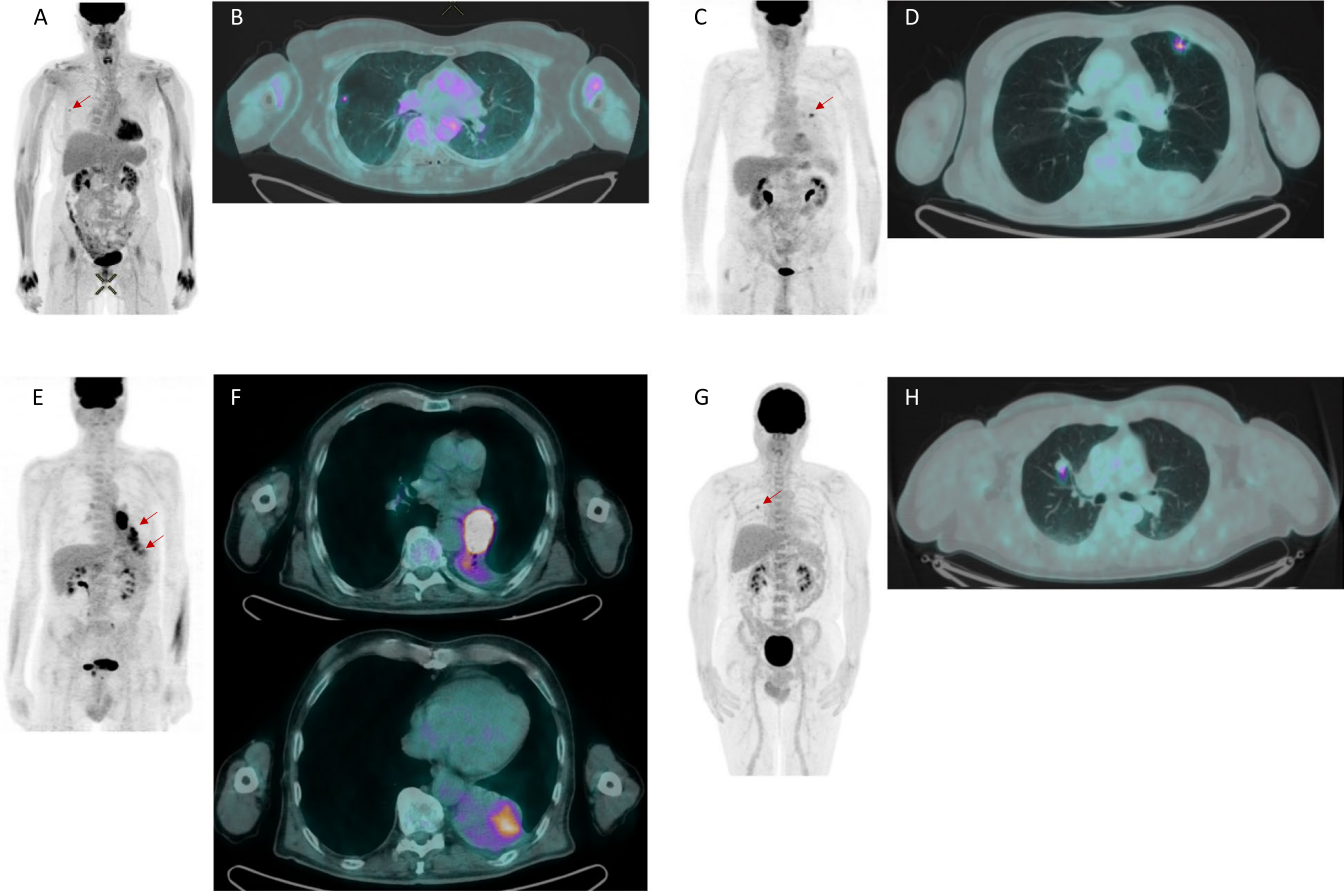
Clinical examples of different pattern of [.^18^F]FDG uptake in patients with suspected lung nodules. MIP and axial fused PET/CT of four different patients diagnosed with a benign intraparenchymal lymph node (a, b), primary lung adenocarcinoma (c, d), primary lung squamous cell carcinoma (e, f), and a lung metastasis from melanoma (g, h)
